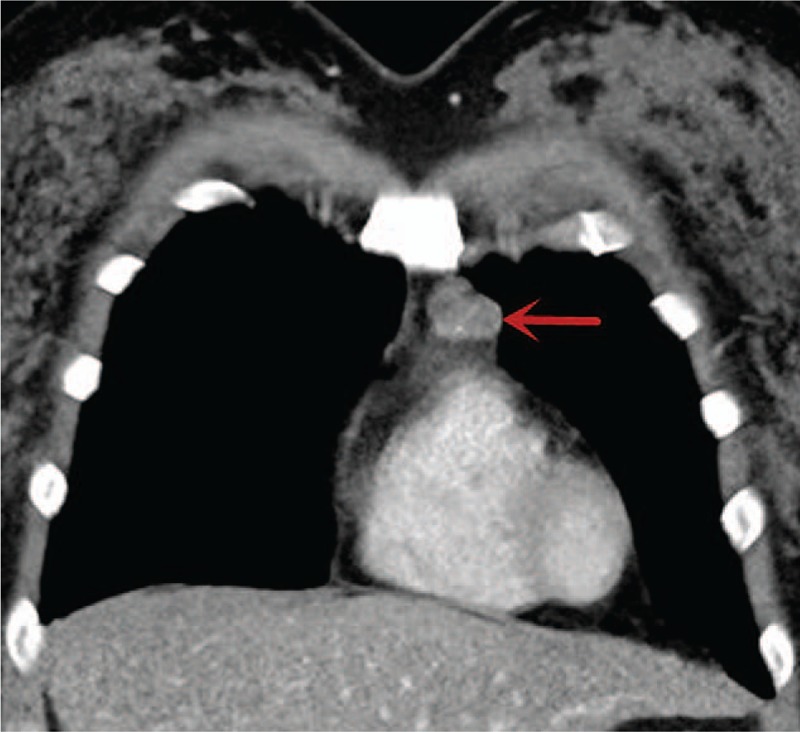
A chest CT scan revealing an oval soft tissue mass measuring 2 × 1.7 cm in size (arrow).
